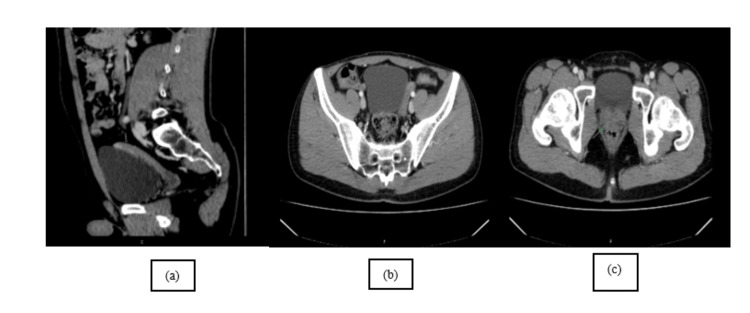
Sagittal venous phase (a) and axial arterial phase (b) CT scans demonstrate a smooth contour, macronodular, with isoattenuation and minimally enhancing ovoidal mass arising from the pelvis, lateral and superior to the bladder. Axial arterial phase (c) CT scan obtained lower demonstrates the presence and normal anatomy of the prostate gland. This figure is the original work of the authors. Patient consent for the use of the image was obtained, as mentioned in the patient consent form.
